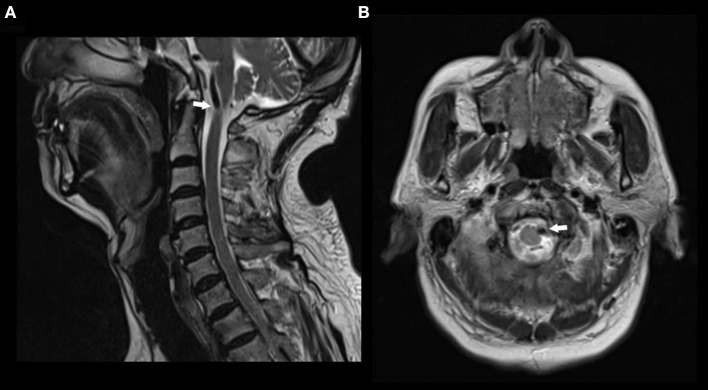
MR images in a patient with nape pain and left leg weakness. (A) Sagittal T2 weighted MR image showing a signal void compressing the upper cervical spinal cord at the atlas level. (B) Axial T2 weighted MR image showing anterolateral compression of the spinal cord by left vertebral artery.
